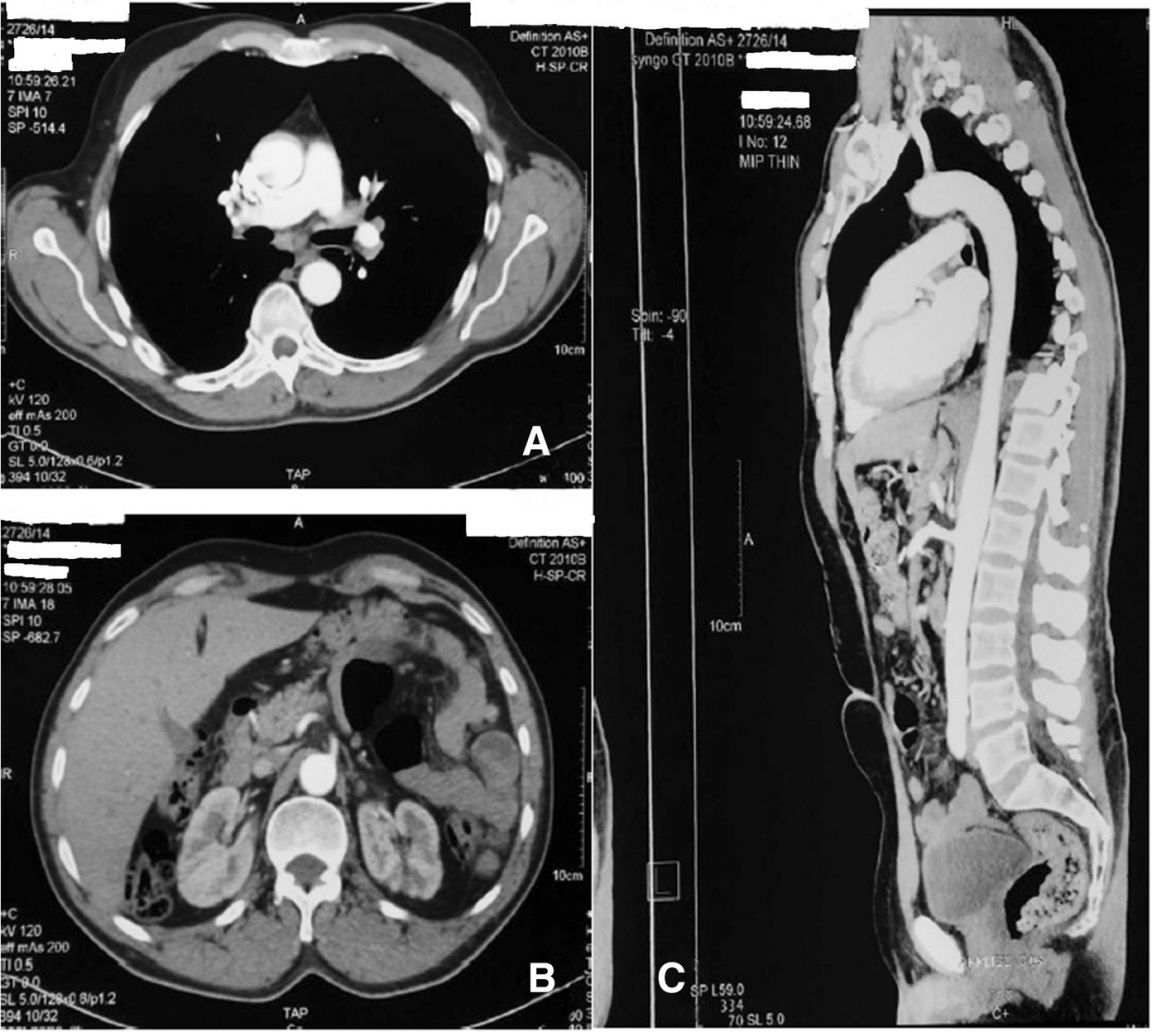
CT angiography showing a complete reversal of the thoracic aorta with disappearance of thrombus. Axial section A and sagittal reconstruction C. Notch sequelae of kidney infarct, axial section B.
